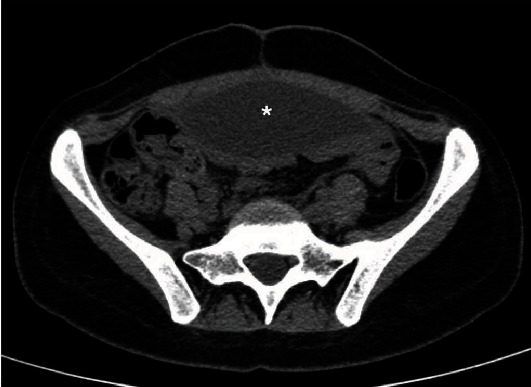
Axial section of the abdominal CT. The asterisk represents the pseudocyst inside the abdominal cavity. This seems to be the cause of the system malfunctioning.
